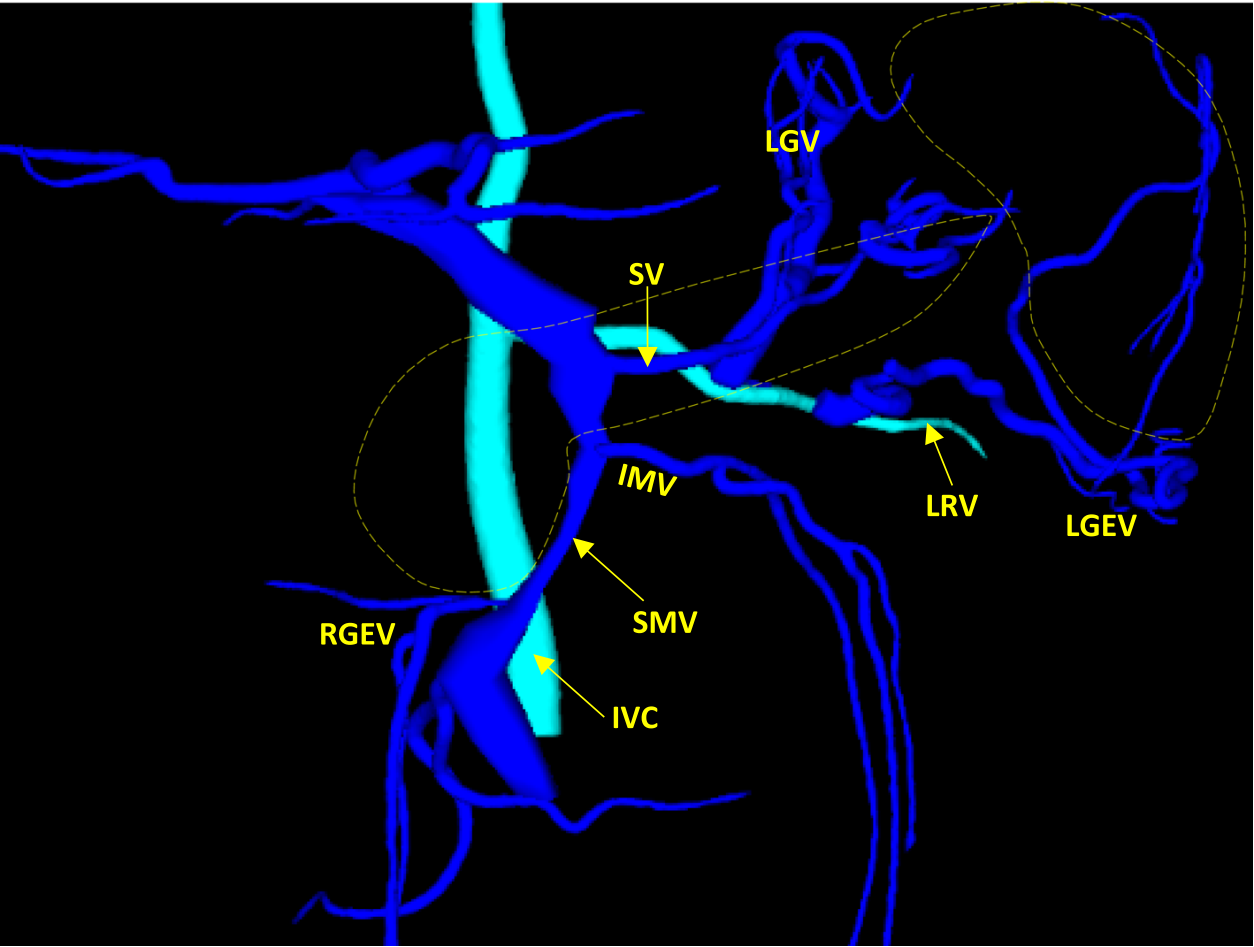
3D-CT. Development of collateral veins was observed due to stenosis of the superior mesenteric vein and the splenic vein. Collateral veins of the left gastroepiploic veins and left gastric veins were partially connected to the right renal vein
